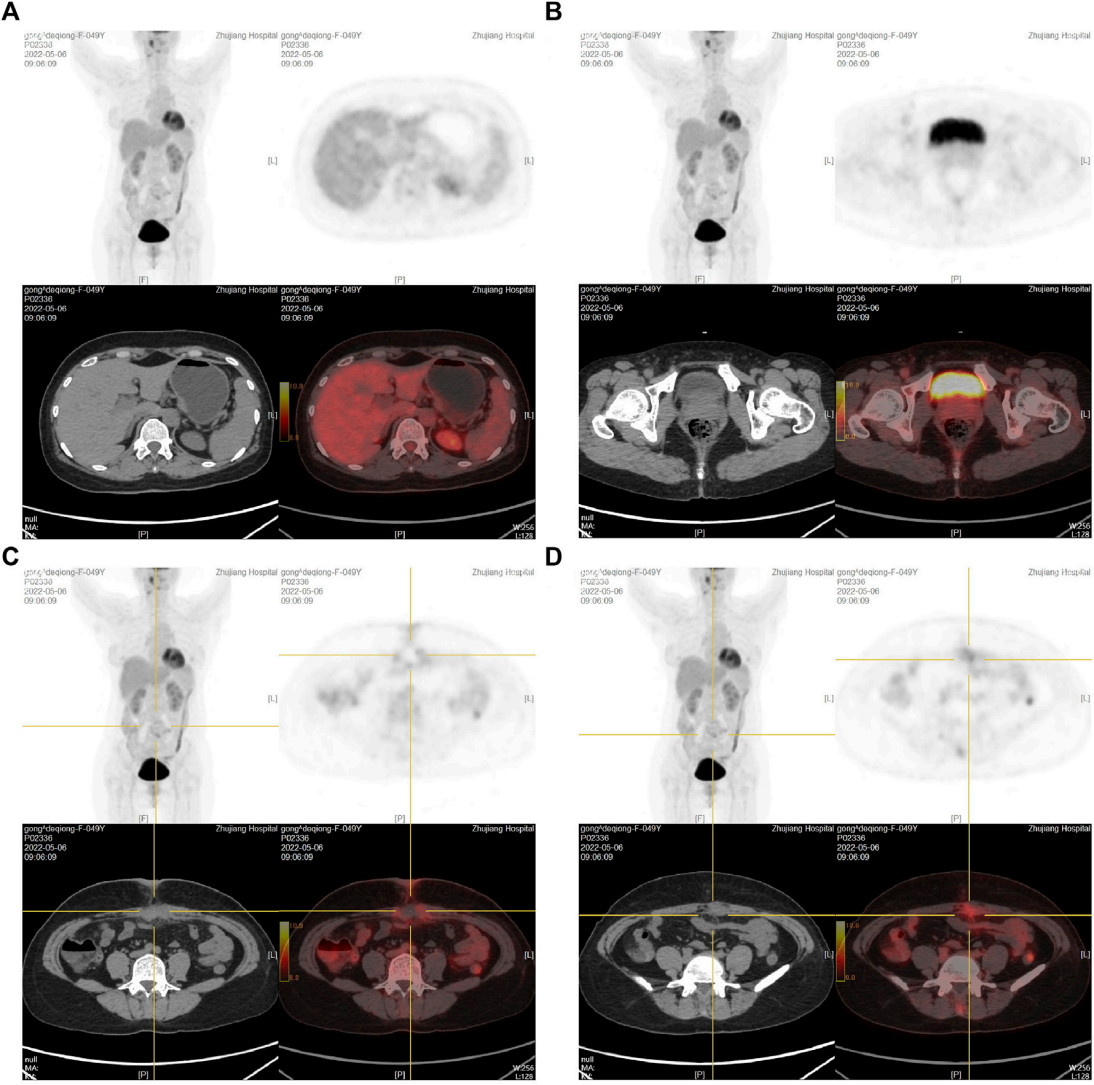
PET-CT examination after the first umbilical nodule resection. (A): PET-CT results at the gallbladder fossa level; (B): PET-CT results at the bladder level; (C,D): PET-CT results at the umbilical nodule level.
